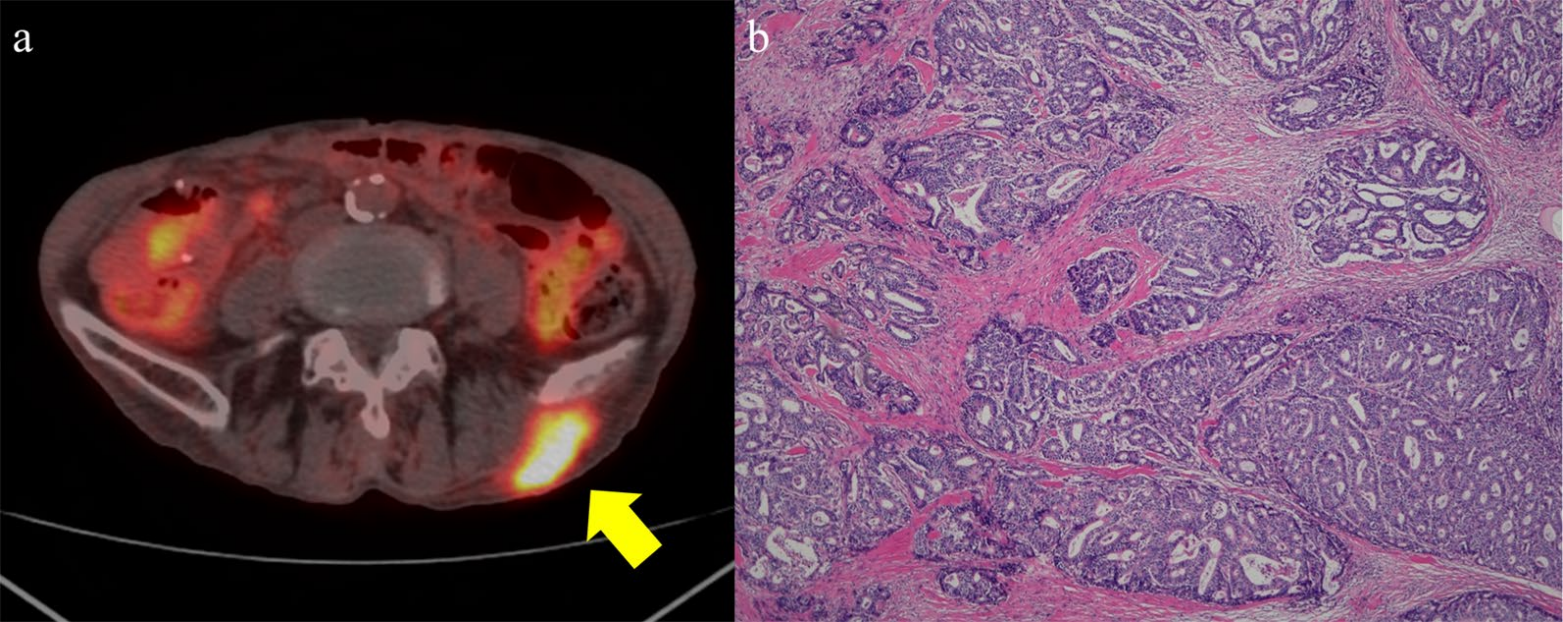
Computed tomography (CT) scan and pathological findings of the third recurrence. a Positron emission tomography (PET)/CT scan shows an elevated FDG intake in the lateral area of the left iliocostalis lumborum muscle. b Pathological examination reveals an adenocarcinoma consistent with metastatic tumor from gastric cancer
